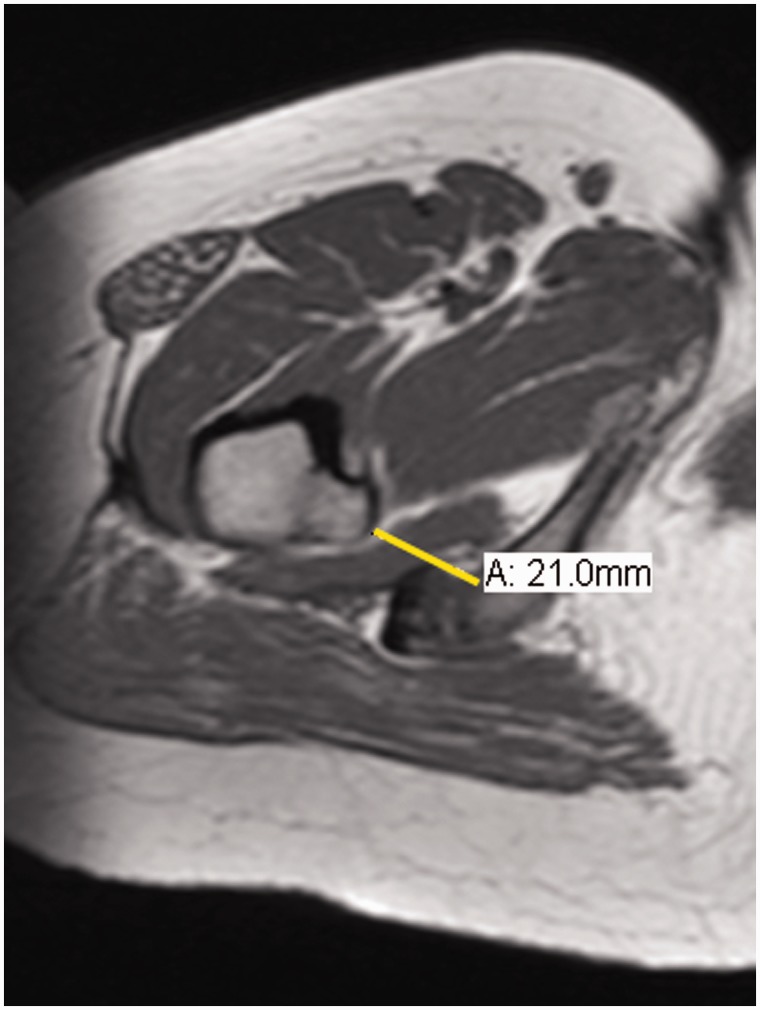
Axial T1-weighted MR image shows typical IFS measurement (line A).
